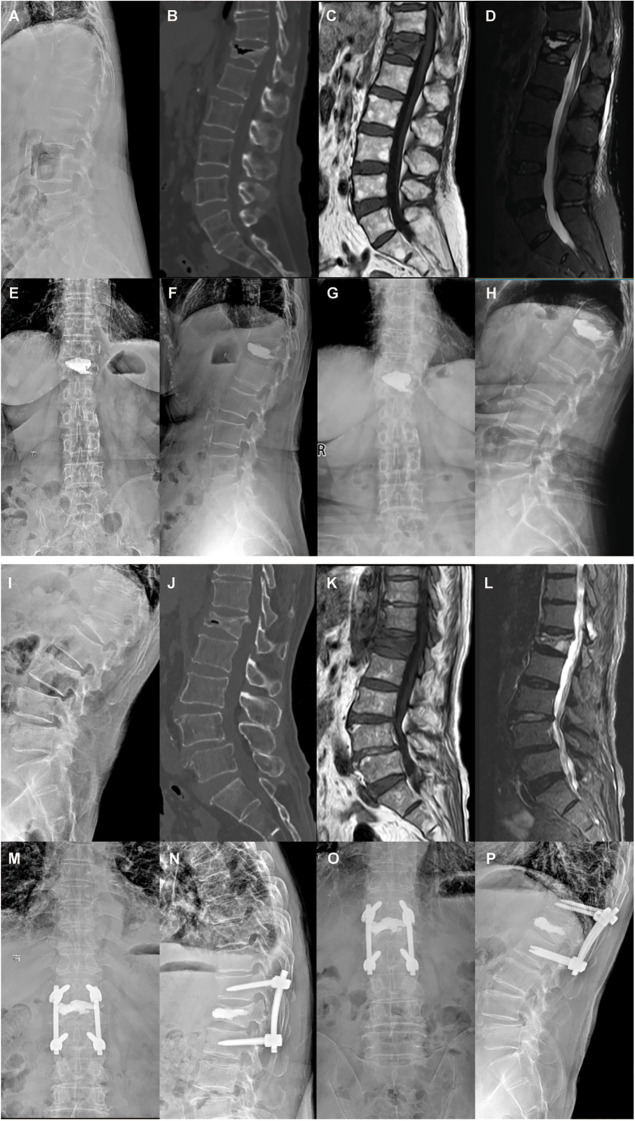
Representative radiographic images of stage III Kümmell disease (KD) without neurological deficits treated with percutaneous kyphoplasty (PKP) or short-segment fixation with vertebroplasty (SSF+VP). (A–H) A representative patient treated with PKP. (A–D) Preoperative lateral radiograph, sagittal CT, and sagittal MRI show marked vertebral collapse, intravertebral cleft (IVC) formation, and local kyphotic deformity. (E, F) Postoperative anteroposterior and lateral radiographs showing bone cement augmentation after PKP. (G, H) Final follow-up anteroposterior and lateral radiographs showing the cement distribution and vertebral alignment after PKP. (I–P) A representative patient treated with SSF+VP. (I–L) Preoperative lateral radiograph, sagittal CT, and sagittal MRI show severe vertebral collapse, IVC formation, and local kyphotic deformity. (M, N) Postoperative anteroposterior and lateral radiographs showing posterior short-segment fixation combined with vertebroplasty. (O, P) Final follow-up anteroposterior and lateral radiographs showing maintained vertebral alignment, fixation status, and cement distribution.
